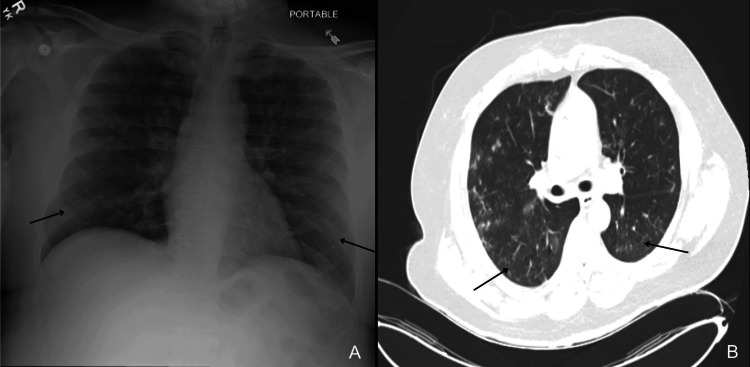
Anteroposterior (AP) chest X-ray (A) demonstrating bilateral pulmonary infiltrates and axial chest computed tomography (CT) scan (B) showing bilateral ground-glass opacities.
